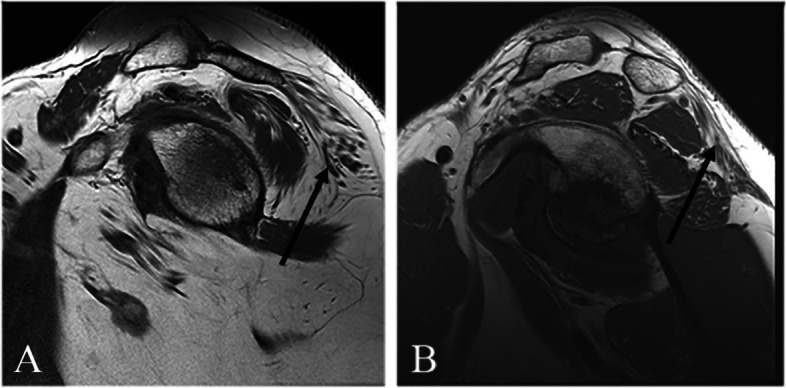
Sagittal T1-weighted MRI demonstrating fatty infiltration of the deltoid muscle in 2 patients
